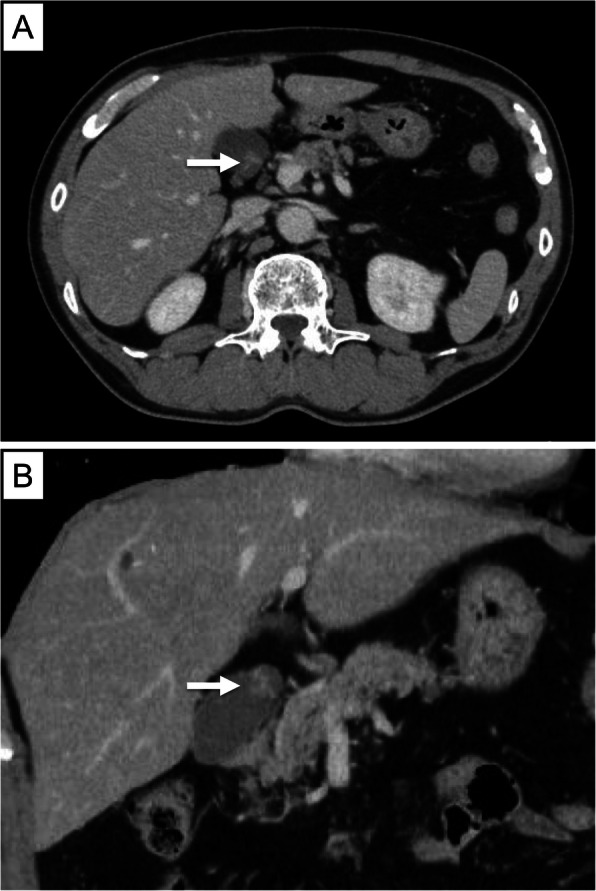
Representative images from the contrast-enhanced CT scan with portal venous phase. Arrow shows a pedunculated polypoid lesion in the neck of the gallbladder (a: axial image, b: coronal image)
